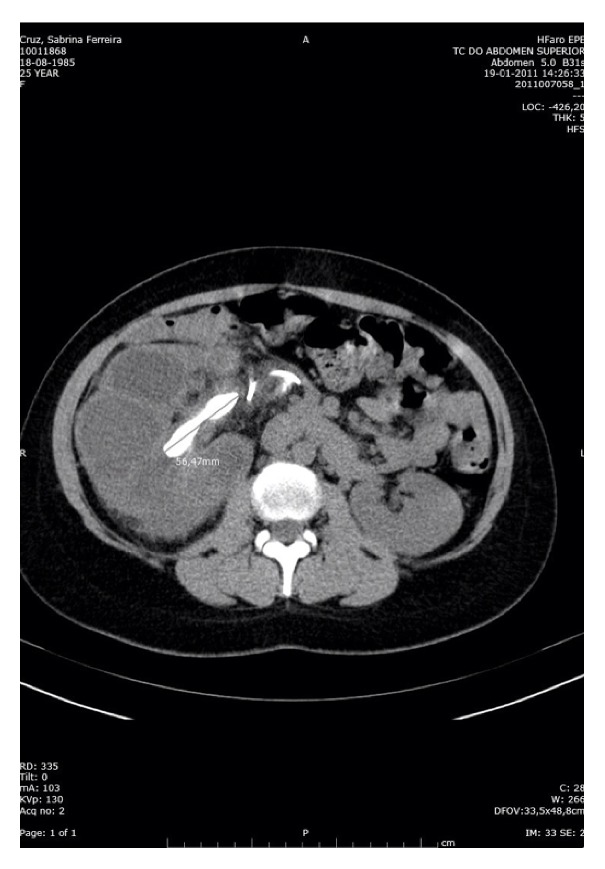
Abdominal pelvic CT showed an enlarged right kidney of 164 mm.
